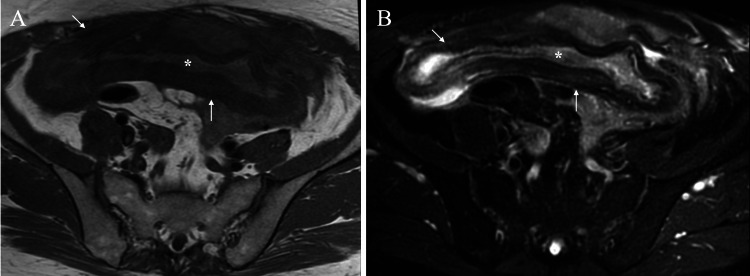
Magnetic resonance imaging (MRI) findings A. T1-weighted images of both the presumed collapsed capsule (arrows) and its internal contents (asterisk) showed low signals. B. Fat-suppressed T2-weighted images showed low signals in the membranous structures (arrows) and high signals in their contents (asterisk).
